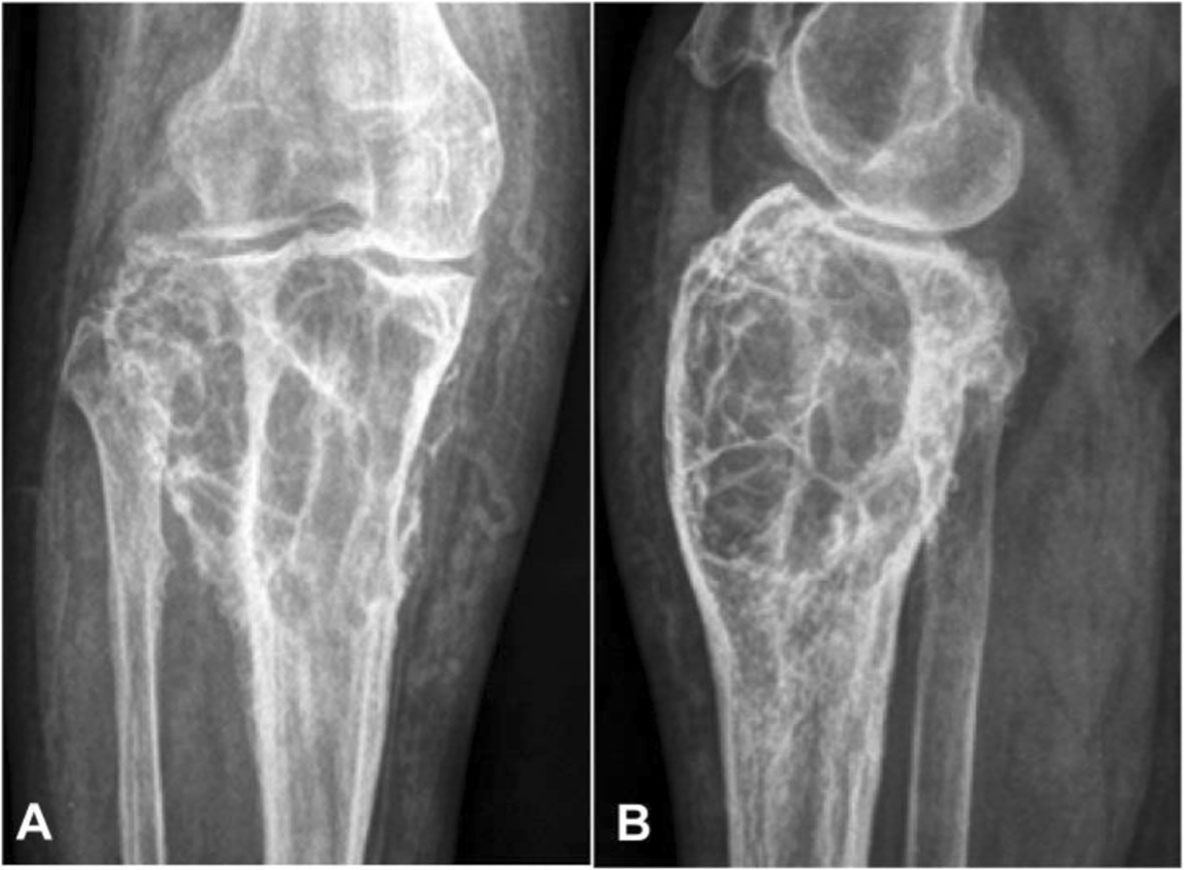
Medullary hemangioma in the proximal end of tibia. Plain radiograph of the anteroposterior (a) and lateral view (b) of the proximal tibia and fibula showed an eccentric, expansile, osteolytic lesion at the proximal end of the tibia, with coarse bone ridges inside the lesion like a honeycomb. The cortical bone was thinned, periosteal reaction was presented, and tortuous vascular structures were seen in the interior soft tissue
